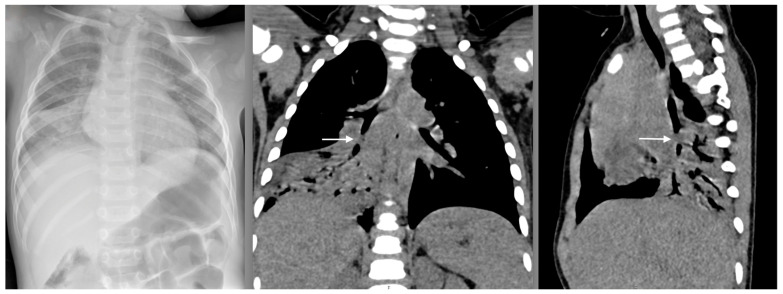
The initial X-ray finding of partial atelectasis of the middle right lung lobe in a 22-month-old boy (left). A CT scan revealed complete obstruction of the intermediate bronchus with a foreign body (peanut) (middle and right) that was successfully removed by rigid bronchoscopy.
